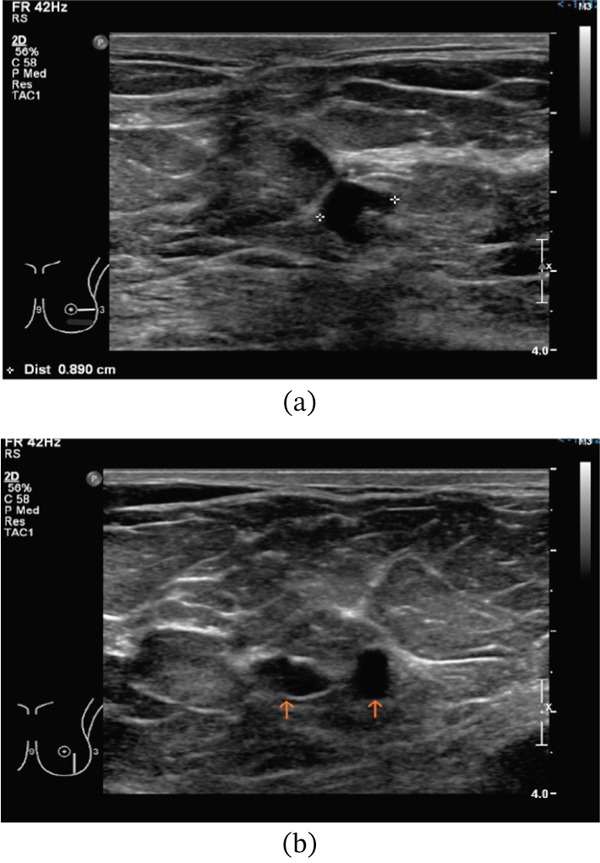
Ultrasound of the lateral left breast in (a) radial and (b) antiradial projections demonstrates (a) an approximately 9‐mm complex cystic/solid mass at the 3 o′clock position (white asterisks) and (b) two adjacent solid masses at the 4‐5 o′clock positions, measuring approximately 9 mm and 6 mm (orange arrows). BI‐RADS 4 (suspicious; biopsy recommended).
